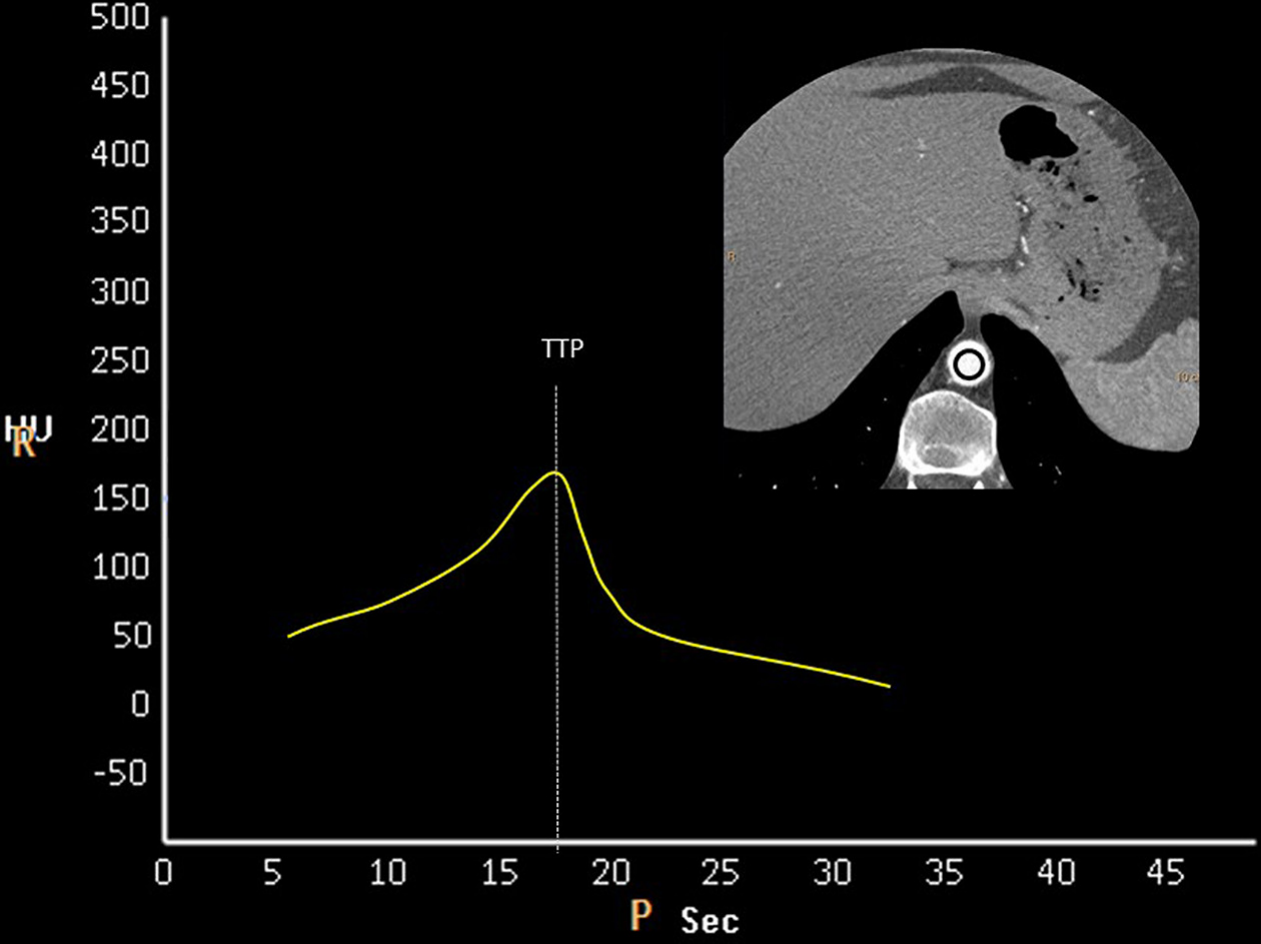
The timing bolus is used to determine the TTP of the descending thoracic aorta. Therefore, in this case study, we synchronized our CTA acquisition when we reached peak contrast opacification in the descending thoracic aorta which accounts for maximal filling of the RCA, dual LAD and LCX. CTA, CT angiography; HU, Hounsfield units; LCX, left circumflex artery; LAD, left anterior descending; RCA, right coronary artery; TTP, time to peak.
